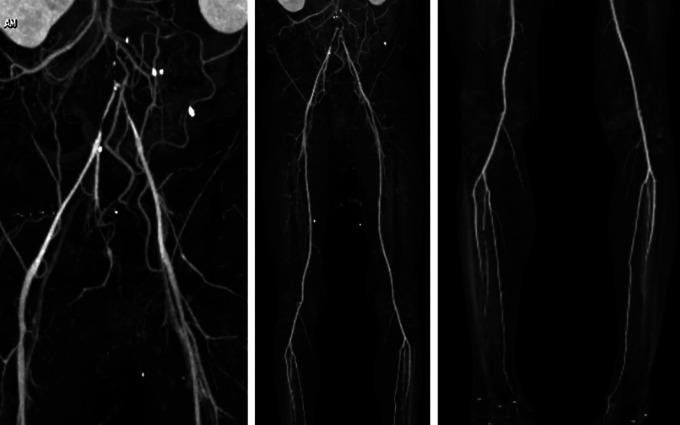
Pre-procedural CT scan angiography.
